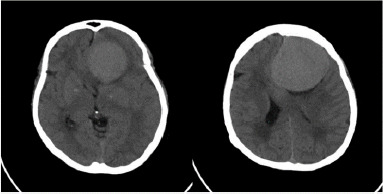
The brain CT showed a hyperdense mass lesion in the frontal lobe with significant oedema
